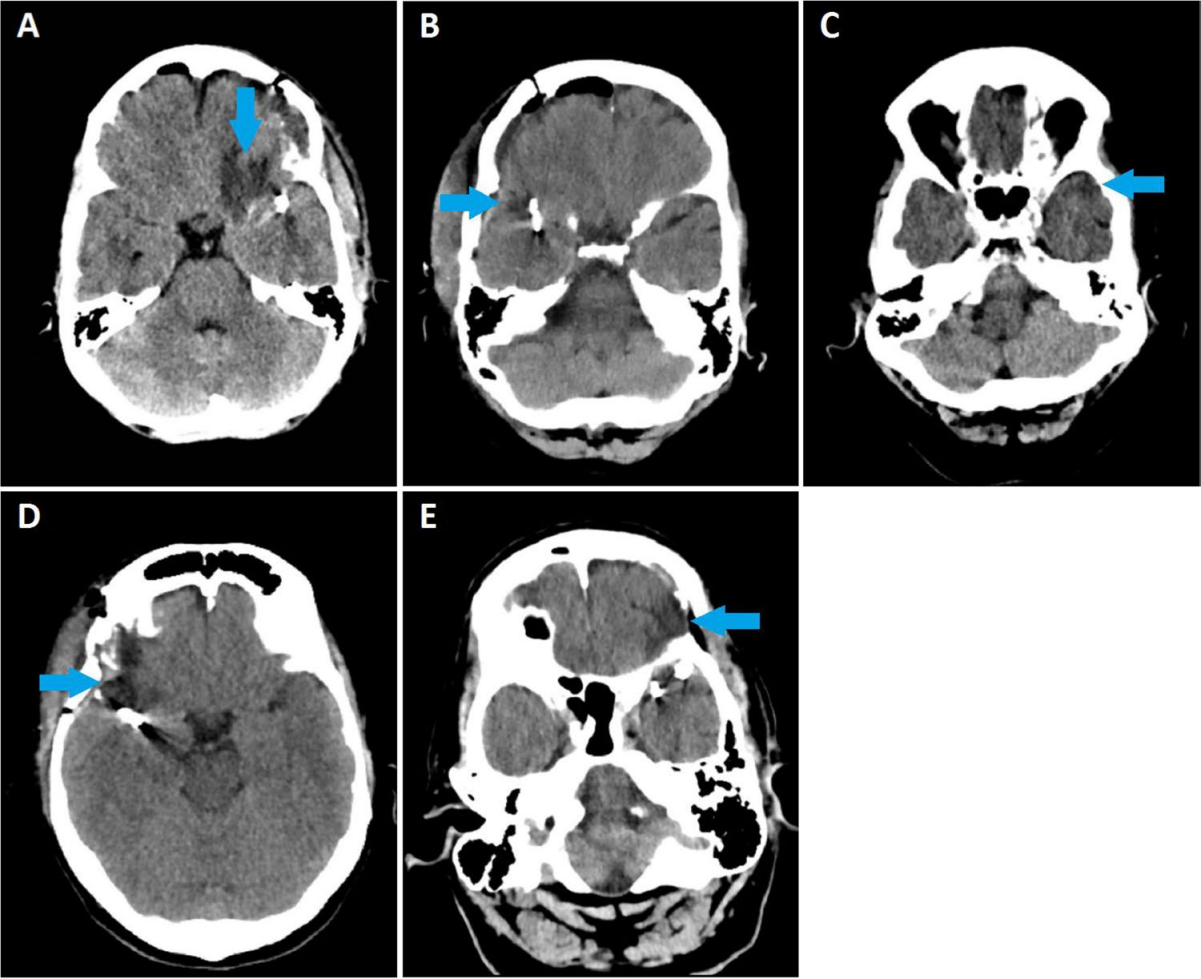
A–E panels of 5 CT scans (A–E). Arrows point to BRI. A CT scan of a severe case of BRI after clipping of a large MCA aneurysm. B–E Examples of CT scans with minor BRI
